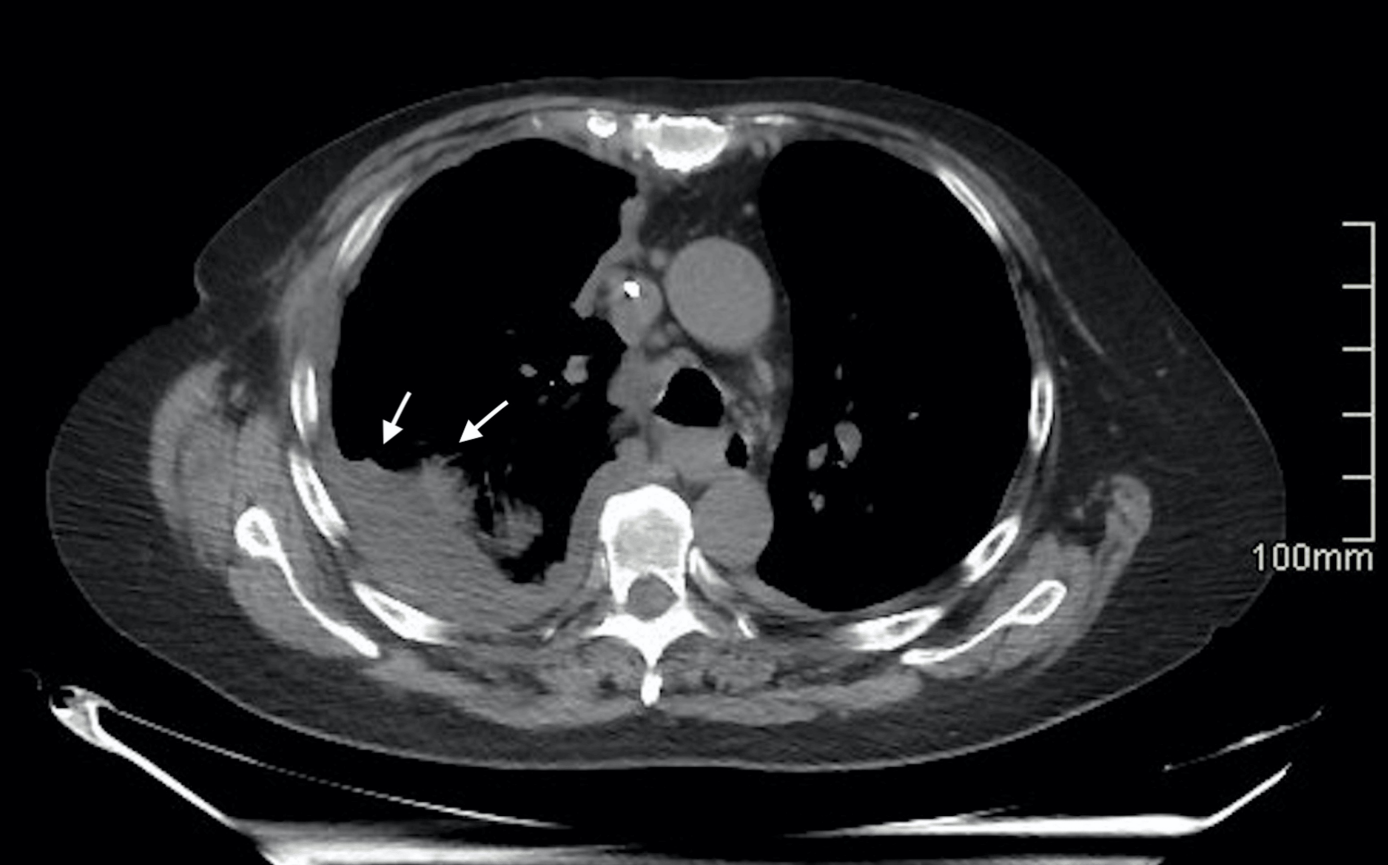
A non-contrast thoracic CT scan identified diffuse tumoral thickening of the pleura in the right hemithorax
